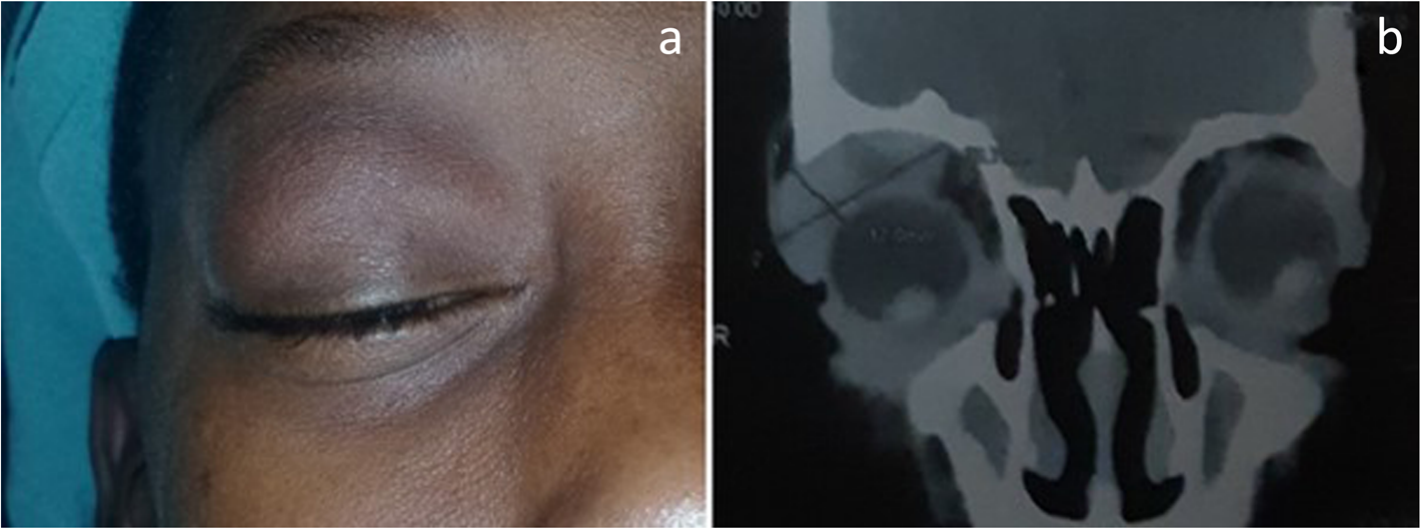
a orbito-palpebral mass, pushing the eyeball slightly downward and inward, with grade 1 exophthalmos (patient under GA). b Computed tomography: Right lacrimal gland mass measuring 29 × 13 mm with low tissue density and heterogeneity that was enhanced by iodine contrast
